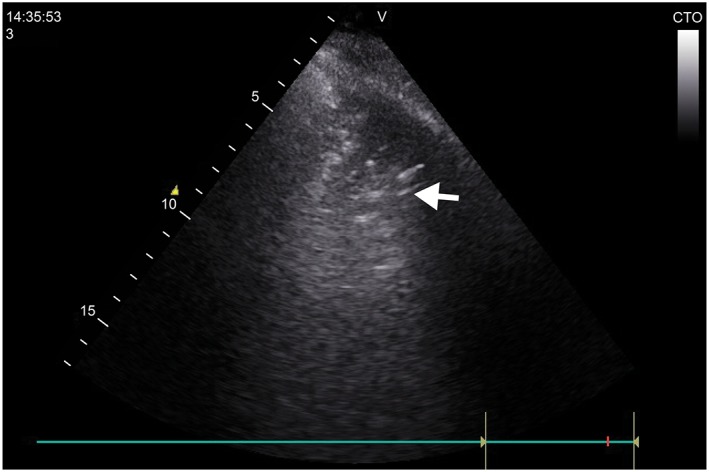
Ultrasound image. A consolidated right lung on ultrasound in a case of pneumonia
